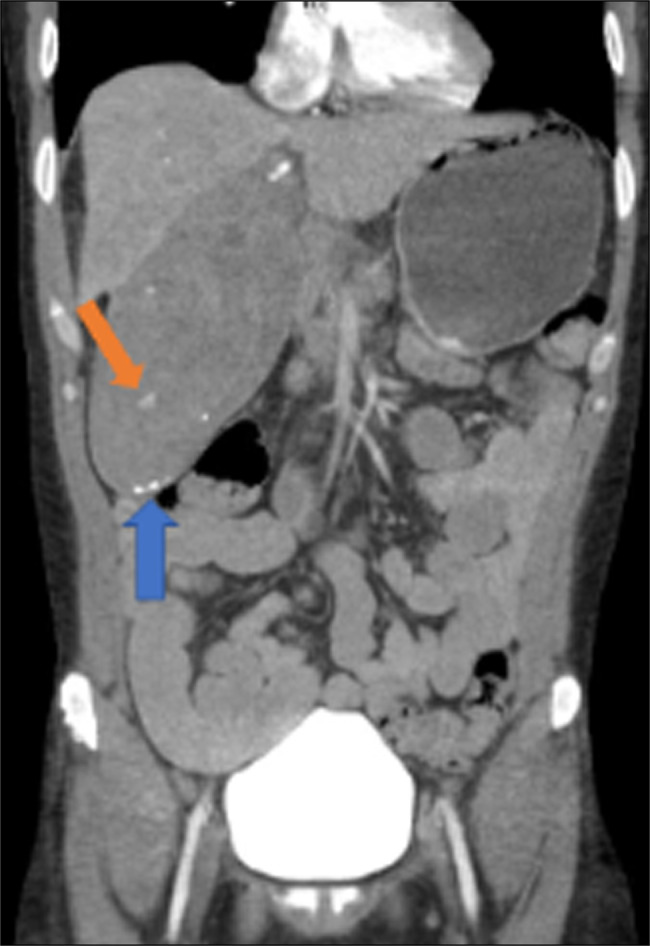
CTA in coronal view of the abdomen showed gallbladder filled with hematoma at the blue arrow and focus of hemorrhage at the orange arrow.
